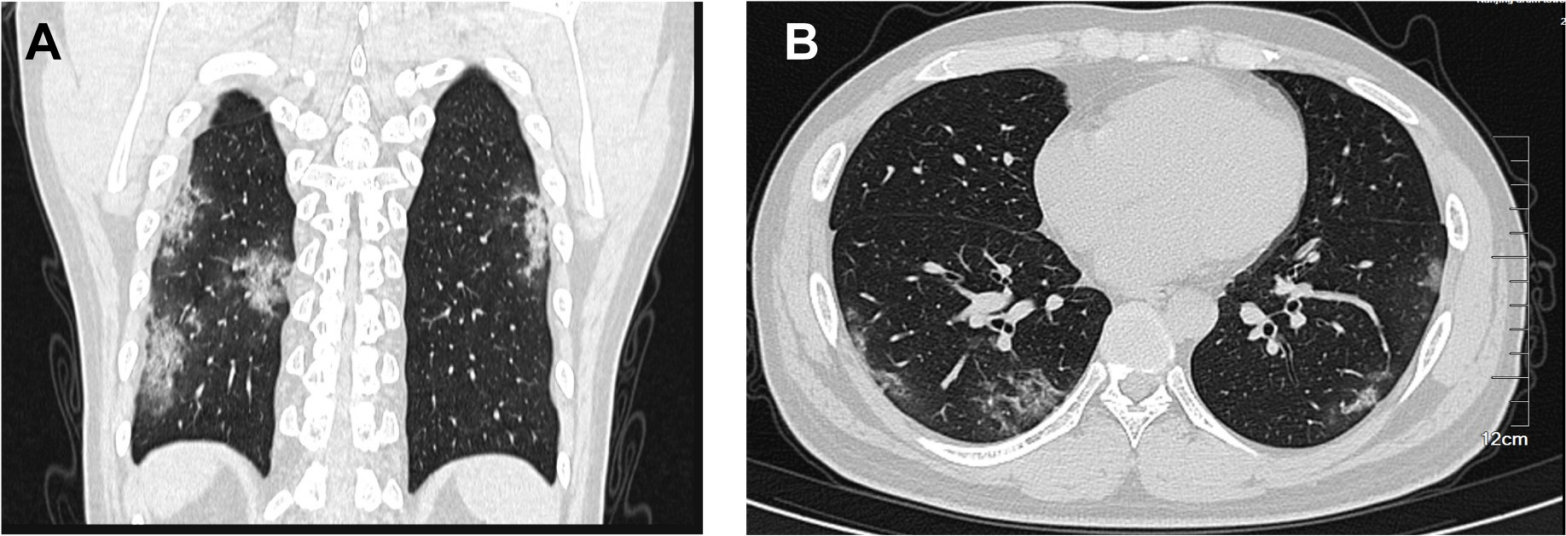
Chest computed tomographic (CT) images of a 30-year-old patient with coronavirus disease 2019 on admission. The chest CT showed bilateral ground-glass opacities in the both lungs.
